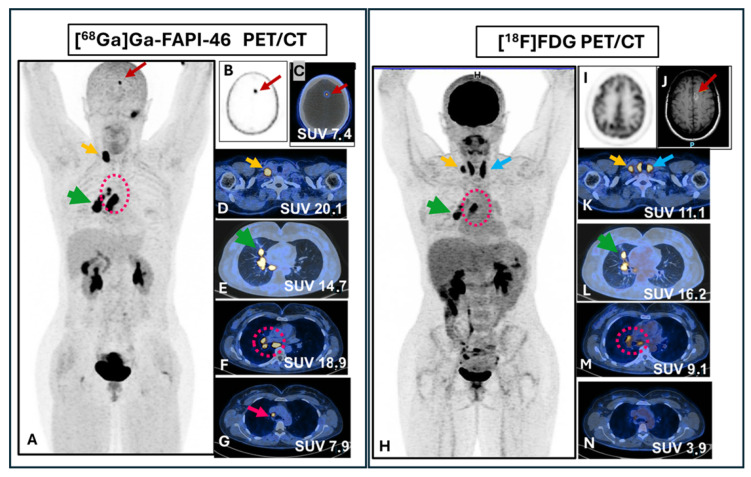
[^68^Ga]Ga-FAPi-46 and [^18^F]FDG PET/CT of a 50-year-old woman with lung adenocarcinoma for initial staging. (A–G): [^68^Ga]Ga-FAPi-46 PET/CT. (H–N): [^18^F]FDG PET/CT. (A,H): Maximum intensity projection (anterior view). (D–G): [^68^Ga]Ga-FAPi-46 PET/CT and (K–N): [^18^F]FDG PET/CT axial fusion images. PET/CT shows intense uptake of both (E) [^68^Ga]Ga-FAPi-46 and (L) [^18^F]FDG in the primary tumor in the right lung (green arrows). Tracer uptake is also observed in ipsilateral pulmonary hilum and subcarinal lymph nodes metastases (pink circles and arrows) and in a right supraclavicular lymph node metastasis (yellow arrow). Of note, PET/CT with [^68^Ga]Ga-FAPi-46 showed greater degree of uptake in mediastinal and supraclavicular lymph nodes (F,G,D) in comparison to [^18^F]FDG (M,N,K). A nodular lesion in central nervous system shown on head MRI ((J), red arrow) is shown on PET/CT with [^68^Ga]Ga-FAPi-46 ((B,C), red arrows) but is not identified on [^18^F]FDG PET/CT (I). Thyroid uptake due to thyroiditis (blue arrow) is observed in [^18^F]FDG PET/CT (K) but not in [^68^Ga]Ga-FAPi-46 images (D).
